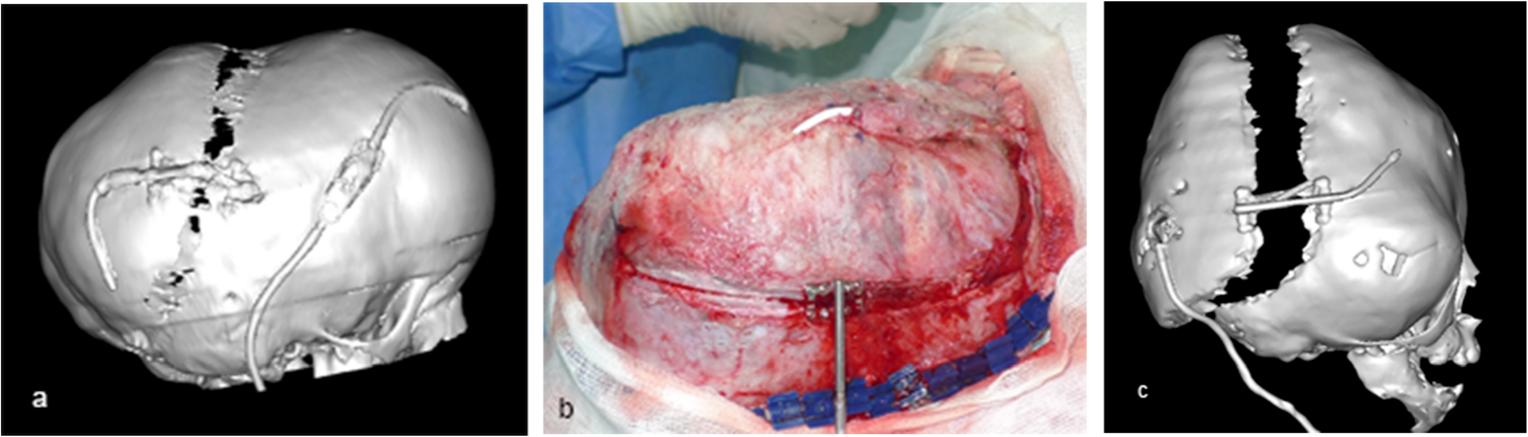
A Placement of the distraction devices when there is a ventriculoperitoneal shunt inserted to the anterior horn of the lateral ventricle. Notice that in some cases the shunt’s subcutaneous part should be relocated to avoid its extrusion during the distraction. b Intraoperative image of the ventriculoperitoneal shunt inserted into the posterior horn, the shunt is left partially unexposed during the procedure. Shown in c is a 3D CT image taken after the completion of the distraction when the shunt moves backwards with the bone flap
